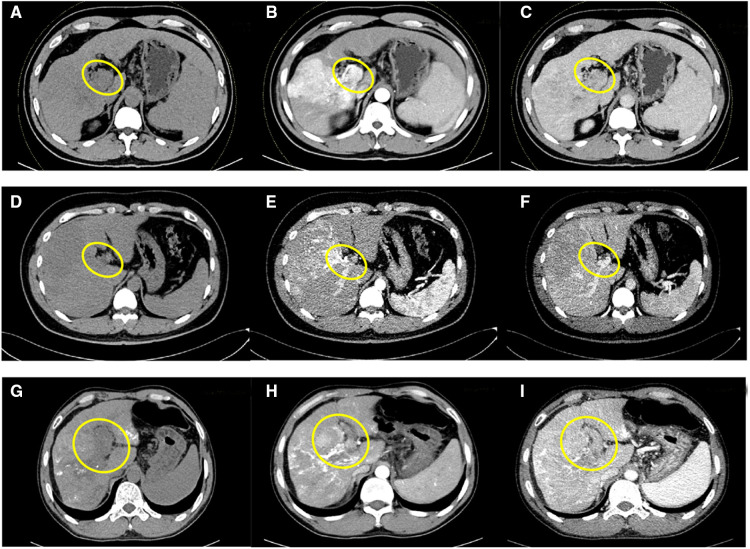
Preoperative CT images of case 1: unenhanced phase (A), arterial phase (B), and venous phase (C); preoperative CT images of case 2: unenhanced phase (D), arterial phase (E), and venous phase (F); preoperative CT images of case 3: unenhanced phase (G), arterial phase (H), and venous phase (I); inside the yellow circle is the portal vein tumor thrombus.
